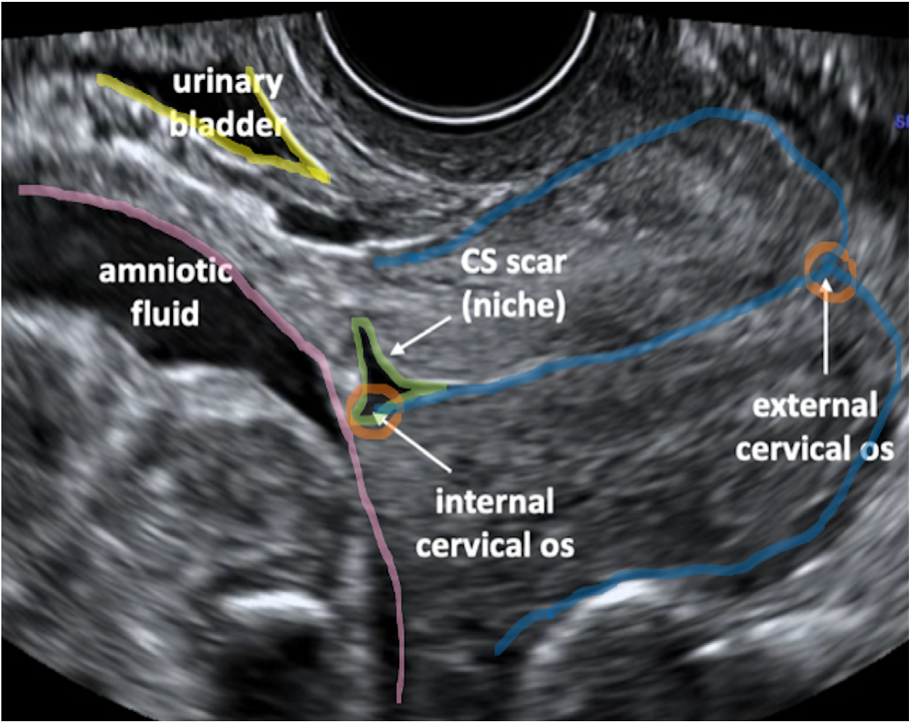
Transvaginal ultrasound image of the cervix and lower uterine segment highlighting the region of interest for 3D volume acquisition.
